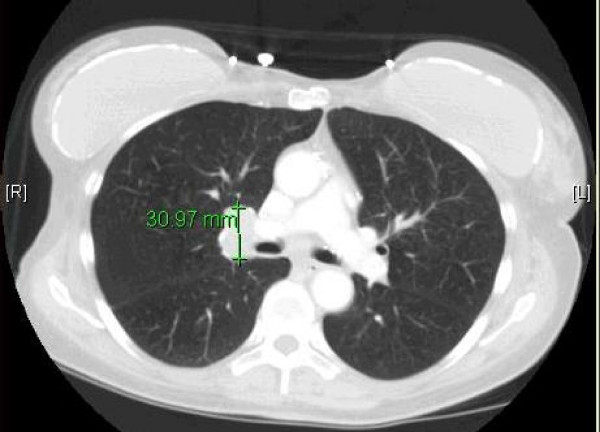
CT scan showing right hilar lymphadenopathy.
